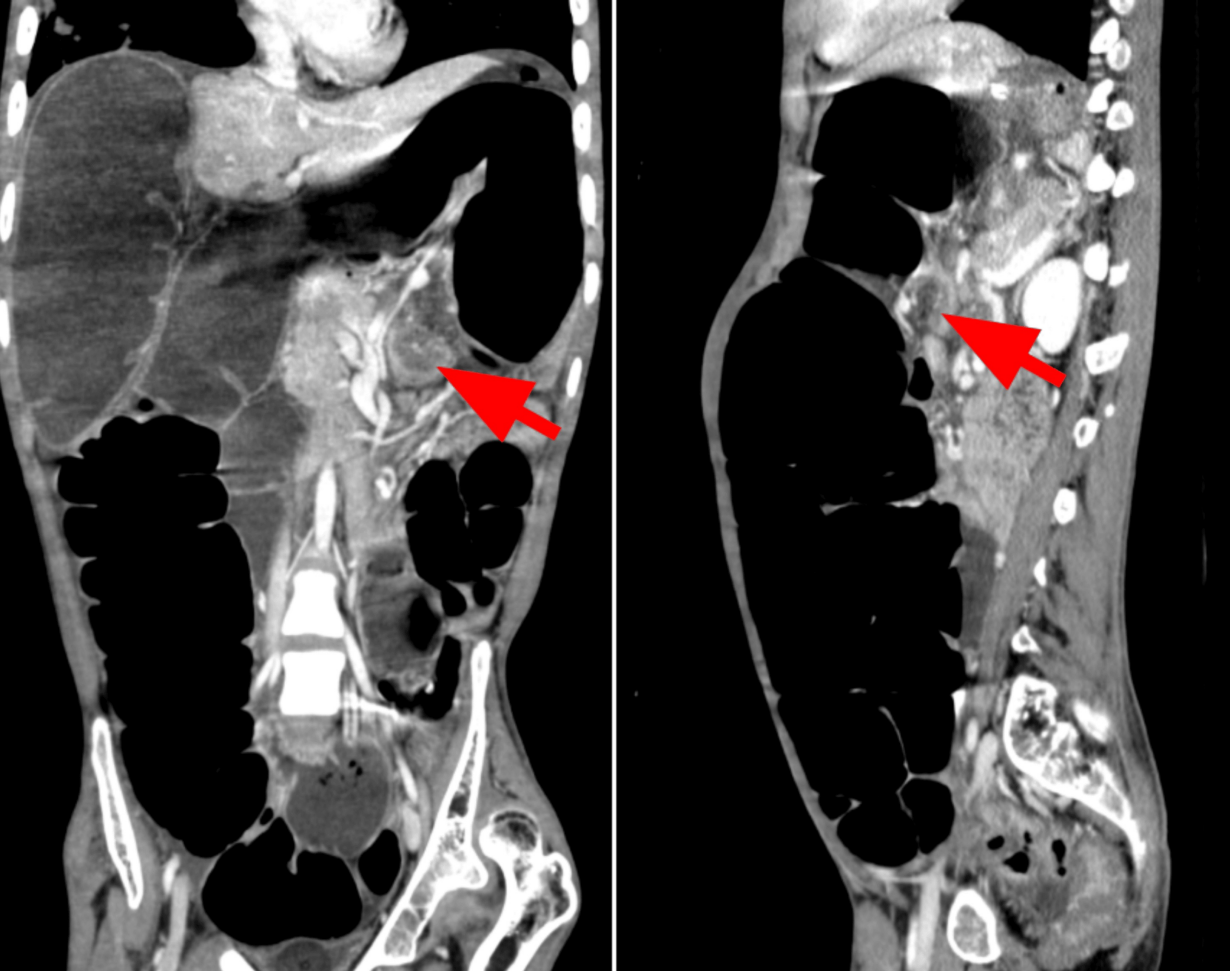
Contrast-enhanced CT of the abdomen and pelvis in coronal (left) and sagittal (right) projections The images show a grossly dilated right hemicolon and redundant transverse colon. A whirlpool sign (red arrow) is visible at the splenic flexure along the AP axis, indicating the transition point. Distally, the descending and sigmoid colon appear collapsed. These CT features confirm bowel obstruction secondary to SFV. SFV, splenic flexure volvulus
